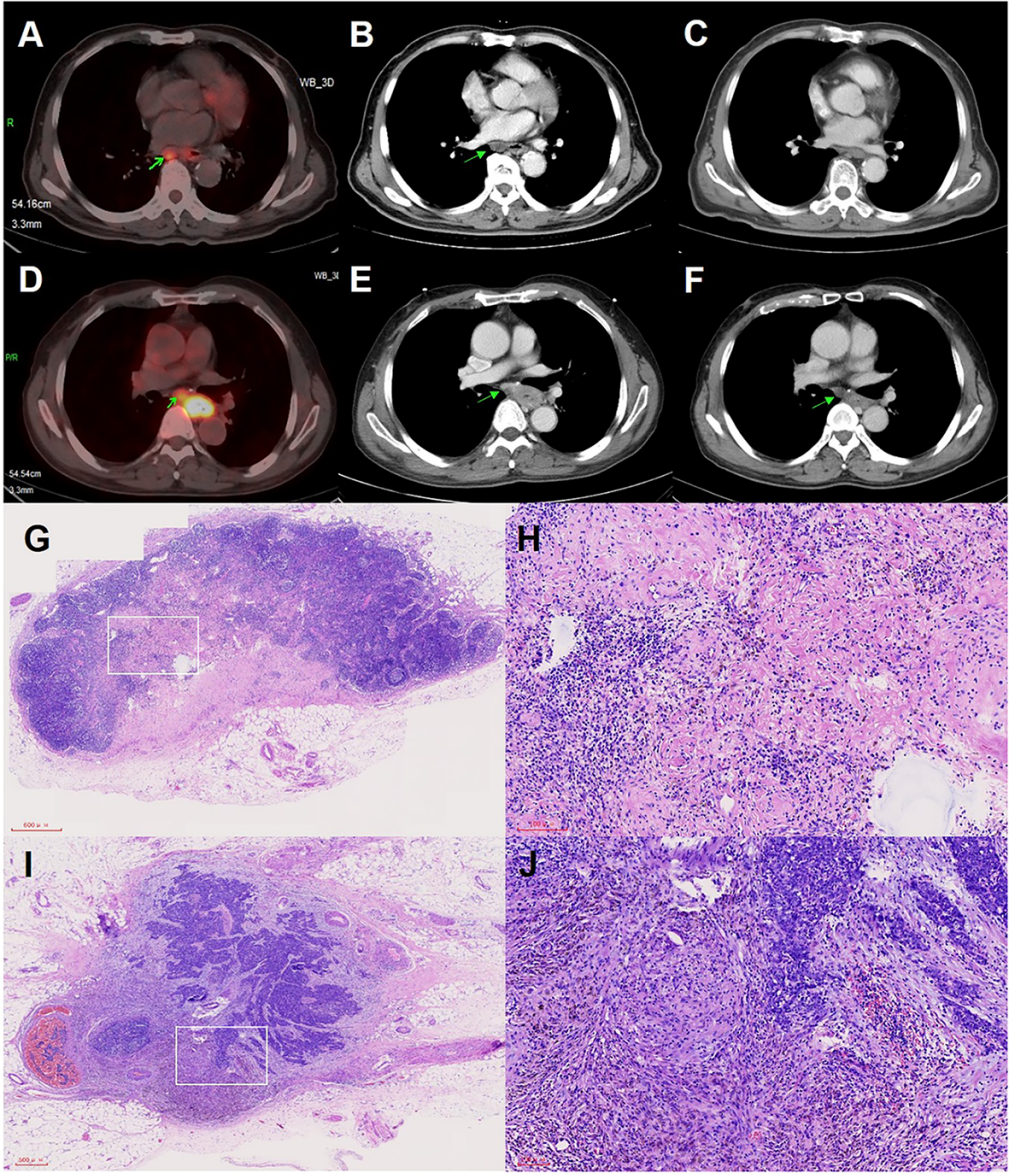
Imaging examination and pathological images of positive lymph nodes (LNs) in pCR status or non-pCR status. (A) PET-CT examination images of positive LNs before neoadjuvant therapy. (B) CT examination images of positive LNs before neoadjuvant therapy. (C) CT examination images of positive LNs of panel B with pCR status after neoadjuvant therapy. (D) PET-CT examination images of positive LNs before neoadjuvant therapy. (E) CT examination images of positive LNs before neoadjuvant therapy. (F) CT examination images of positive LNs of panel E with non-pCR status after neoadjuvant therapy. (G) Pathological images of positive LNs in pCR status. (H) Higher magnification of the white squared area of panel (G, I) Pathological images of positive LNs in non-pCR status. (J) Higher magnification of the white squared area of panel (I).
